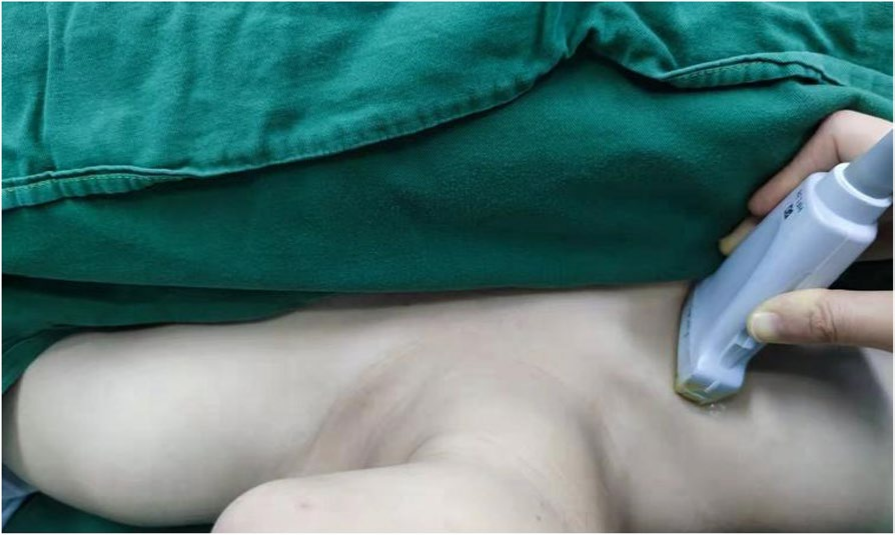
The sonographic location of subclavian vein using the linear array probe
